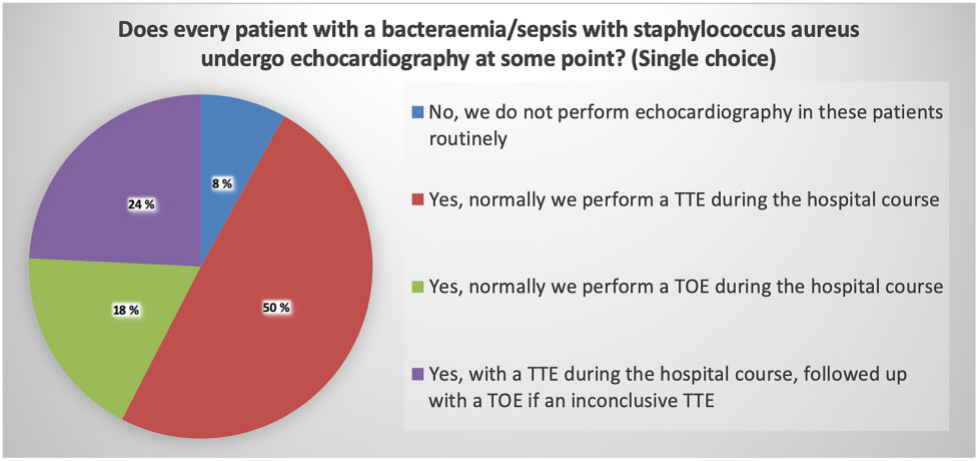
Pie chart showing that most centres perform echocardiographic examinations during a hospital stay in patients with a *Staphylococcus aureus* bacteraemia/sepsis.
